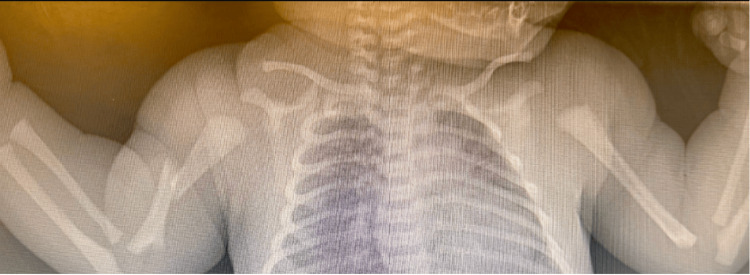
X-ray image after birth Acute appearing fracture of the right mid humeral diaphysis with mild angulation and 1.1 cm of a fracture fragment overlap. No other site of acute osseous trauma.
